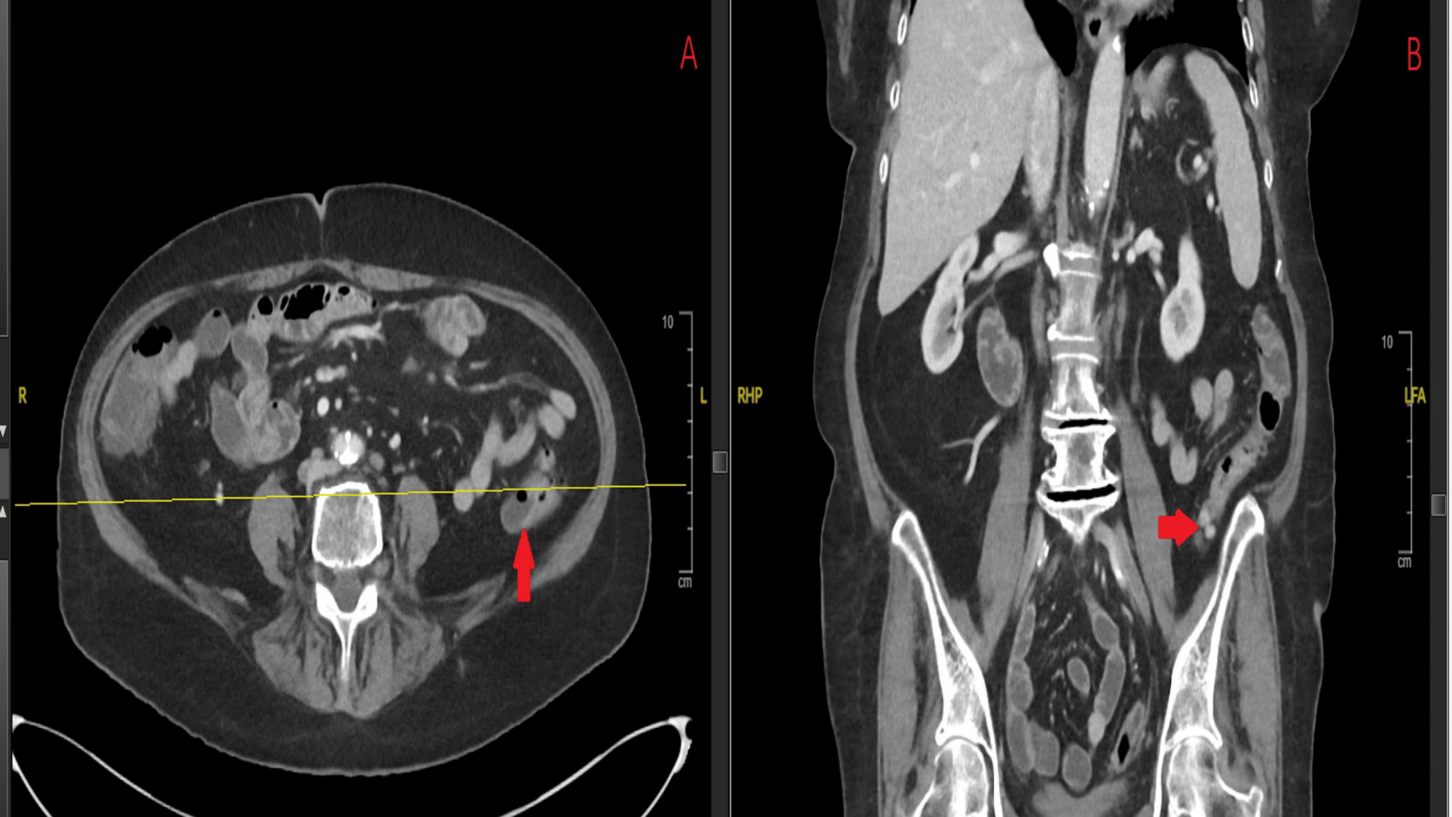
Contrast-enhanced CT of the abdomen obtained during the initial hospitalization demonstrating mucosal thickening of the left colon with pericolonic fat stranding and fluid-filled colonic loops consistent with acute diverticulitis (arrows). (A) Axial view. (B) Coronal view. CT: computed tomography
